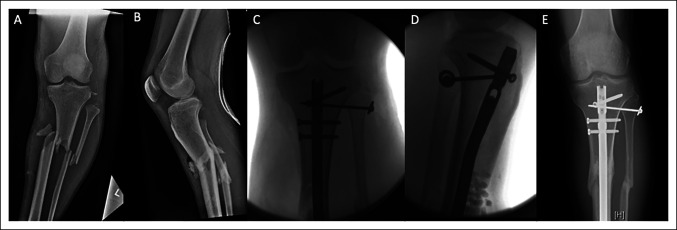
A and B, Original injury radiographs showing a Gustilo-Anderson grade IIIA open left tibia/fibula fracture (OTA classification 42-A3) with associated proximal tibiofibular dissociation. C and D, Intraoperative fluoroscopic images demonstrating excisional débridement of the anterior tibial wound with open reduction and intramedullary nail fixation of the tibial diaphyseal fracture with three proximal interlocking bolts. In addition, an open reduction and internal fixation of the proximal tibiofibular joint was done with 3.5 mm cortical screw and washer. E, Ten-month postoperative follow-up radiograph demonstrating union of the tibial and fibular diaphyseal fractures with maintained reduction of the proximal tibiofibular joint.
